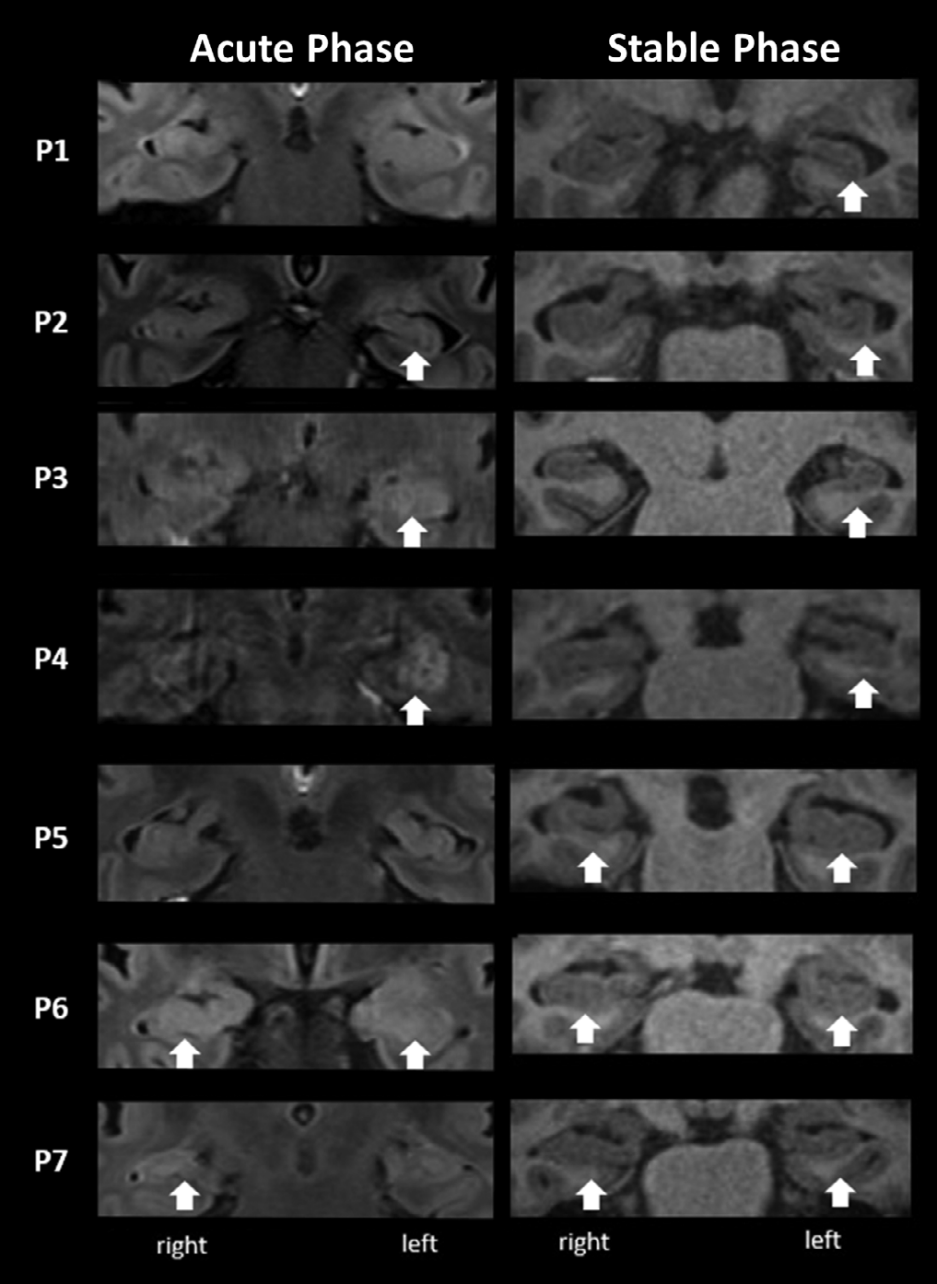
Structure MRI of patients from initial presentation in the acute phase (T2 coronal FLAIR; left column) and at time of neuropsychological testing in the acute phase (T1 coronal; right column). White arrows indicate increased signal intensity in the left column and subsequent atrophy in the right column (as reported by neuroradiologists)
